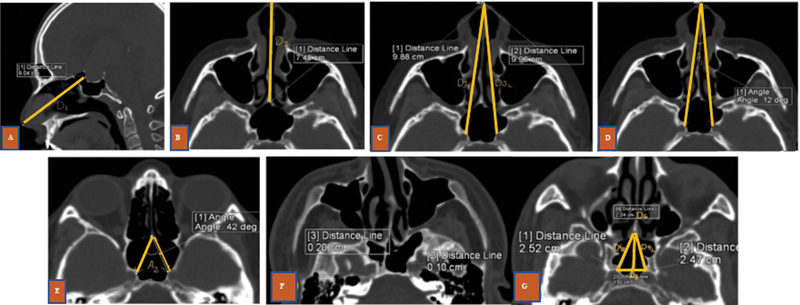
Sagittal window of computed tomography (CT) showing D1 - Distance from the columella to the anterior-thinnest part of sella) (
